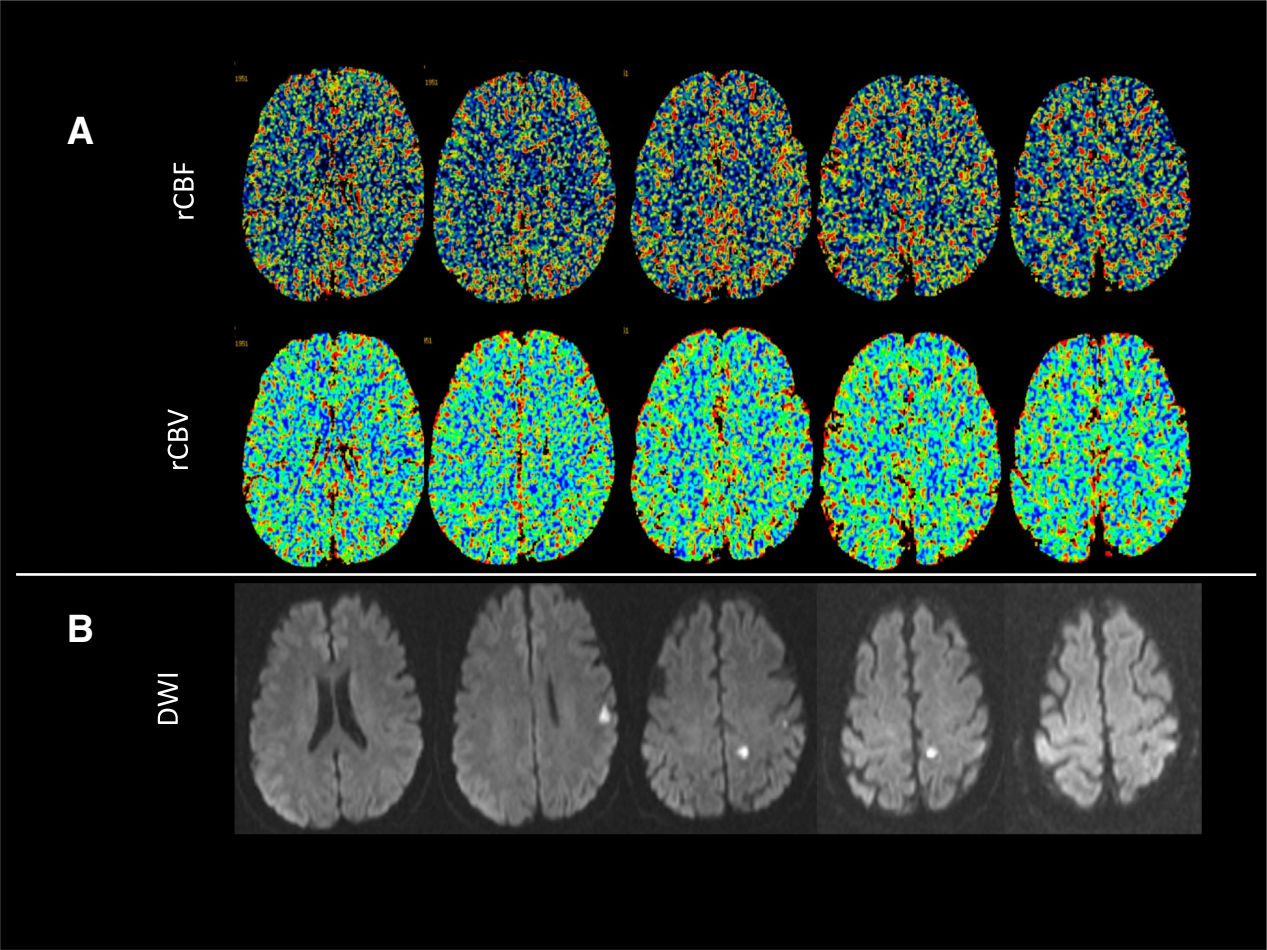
CT perfusion and MR imaging (a) CT perfusion: CBF and CBV show no remarkable difference between the bilateral. Hemispheres. (b) DWI: acute infarcts in the left MCA territory. DWI = diffusion –weighted imaging; MCA = middle cerebral artery; rCBF = regional cerebral blood flow; rCBV = regional cerebral volume
